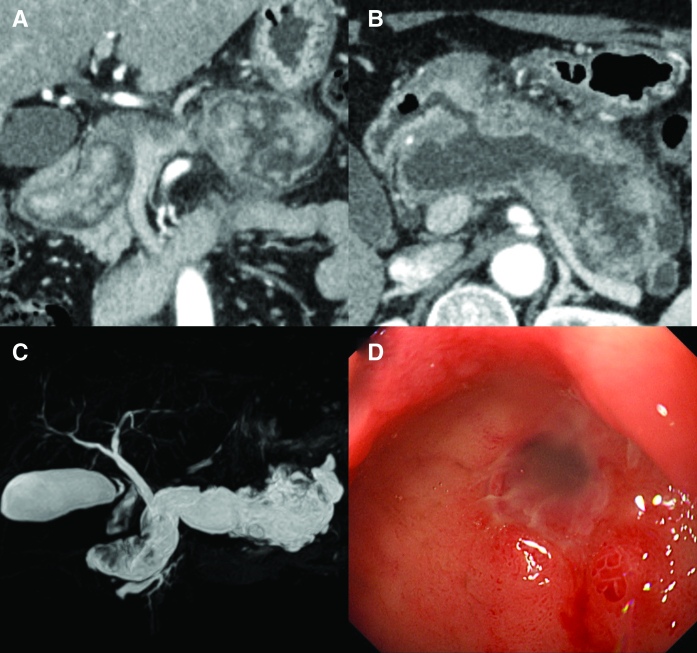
(A) Contrast-enhanced computed tomography of the abdomen reveals an enlarged pancreas with multiple papillary masses in the main pancreatic duct and extensive ductal dilation. (B) Computed tomography shows a fistula between the main pancreatic duct and the duodenum. (C) Magnetic resonance cholangiopancreatography shows an enlarged pancreas with extensive ductal dilation. (D) Esophagogastroduodenoscopy shows a fistula with mucus discharge in the duodenal bulb.
